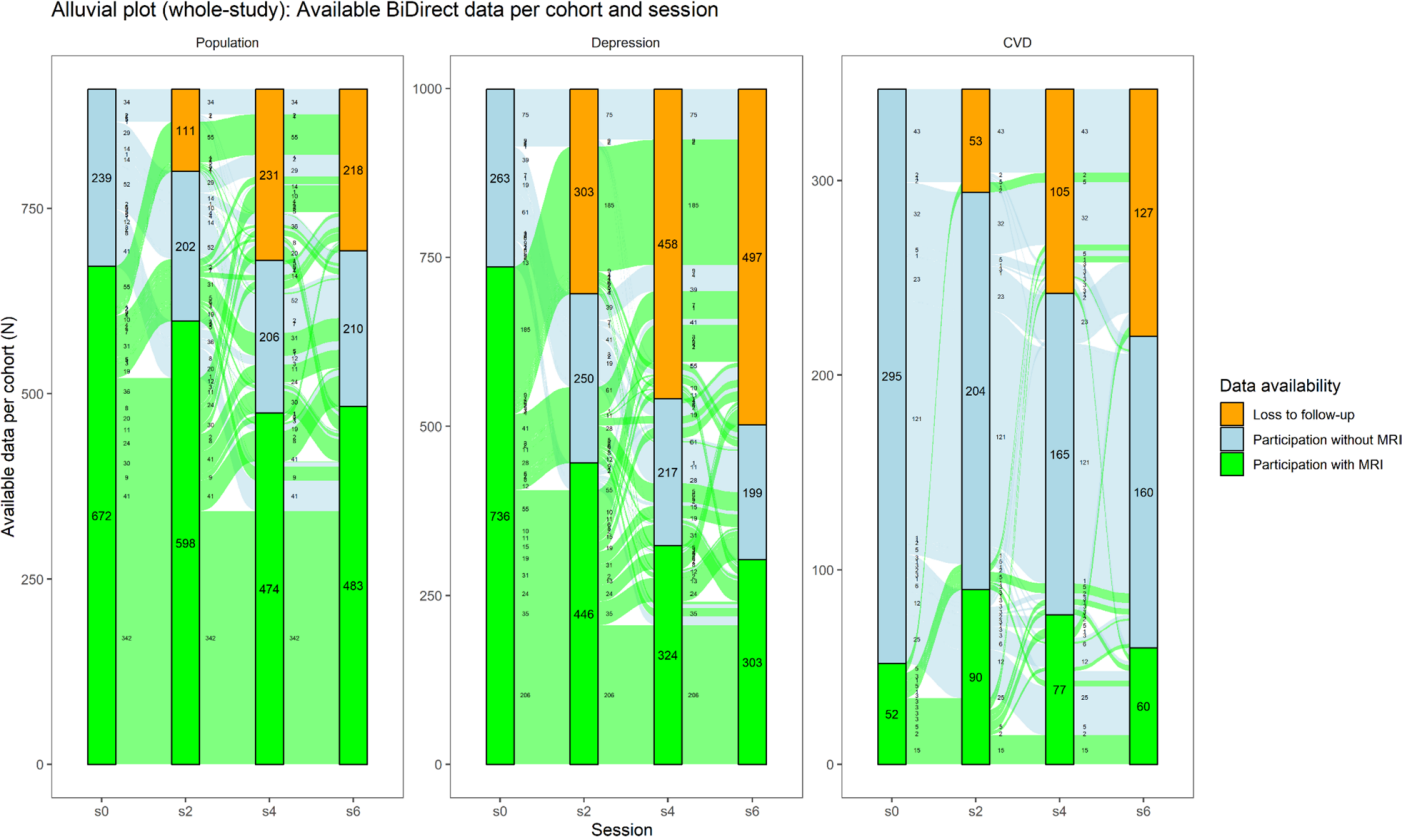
Alluvial plot of available data stratified by session on the *x*-axis and cohort by horizontal subplot. The bars (strata) show the proportion of available or missing data per cohort and session from Table 1 on the *y*-axis. The numbers in the strata show the observations in each category. The alluvia are lines that extend from s0 to s6 and contain the number of observations that fall into each category. The *data availability* coloring of the bars shows loss to follow-up (orange), study participation without acquisition of MRI data (blue), and available MRI data (green)
